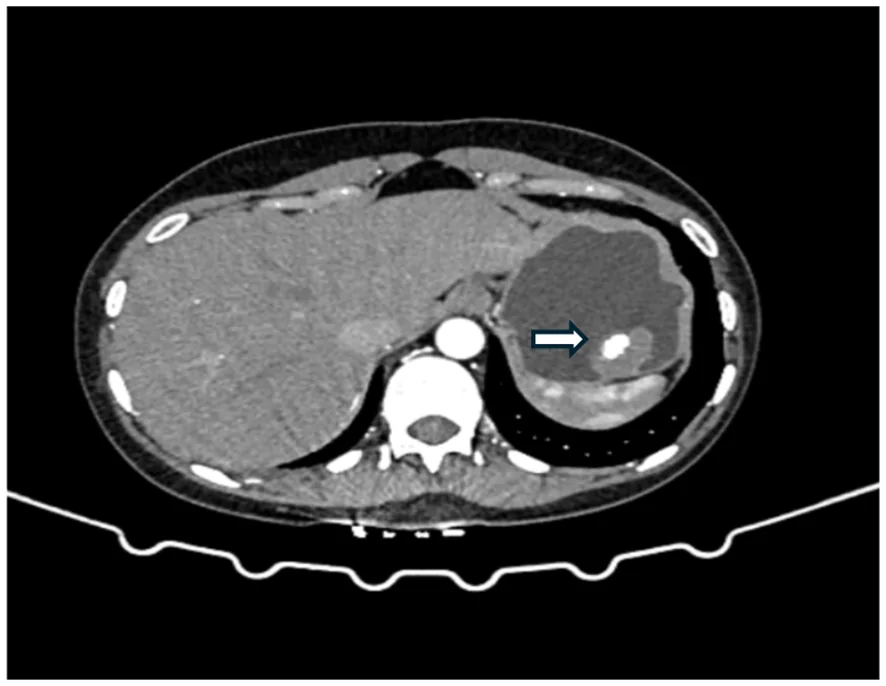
CT shows a high-density nodule with internal calcifications(arrow).
